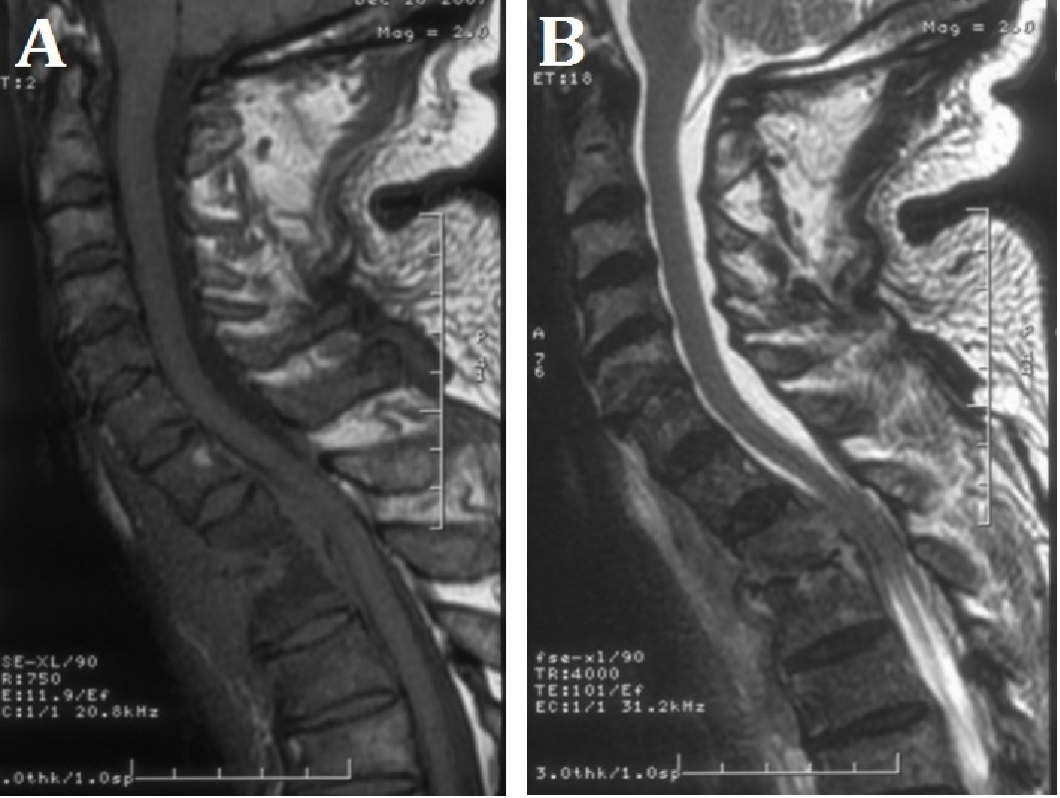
Preoperative MRI Imaging Preoperative sagittal (A) T1- and (B) T2-weighted MRI showing discitis and osteomyelitis at T1 and T2, anterior epidural collection extending from C7-T2, and severe canal stenosis and cord compression.
